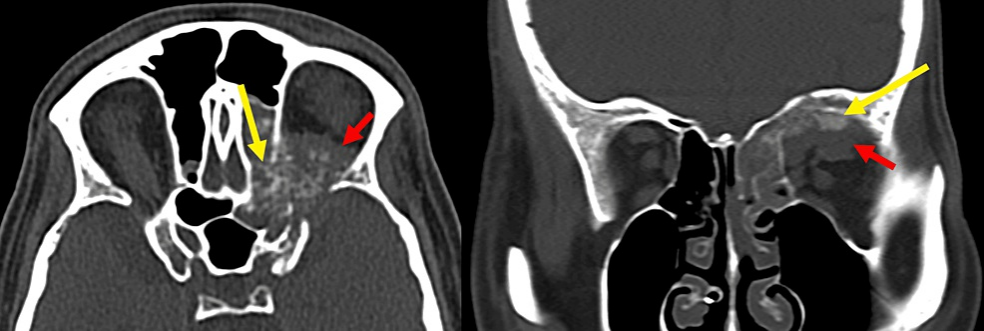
CT head without contrast: Left orbito-ethmoid expansile and lytic osseous mass with aggressive periosteal reaction (yellow arrow) and soft tissue component (red arrow). The mass involves the orbital apex and destroys the bony optic canal.
